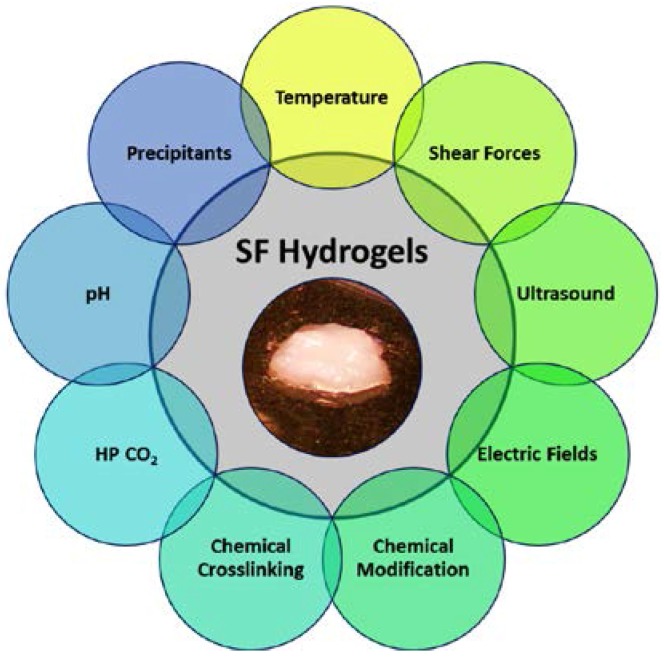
SF hydrogels can be prepared from several methods. Chemical methods: precipitants, pH, HP CO_2_, chemical crosslinking, chemical modification. Physical methods: temperature, shear force, ultrasound, electric fields.
